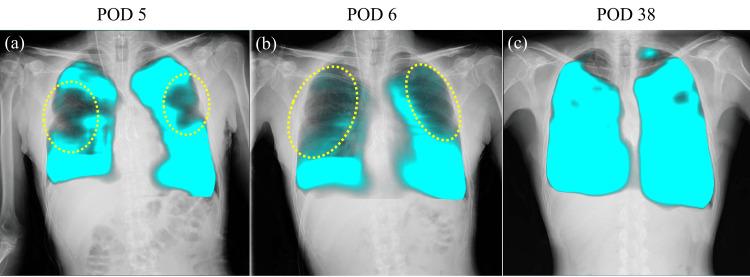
Patient’s dynamic images obtained using dynamic digital radiography (DDR) (PL-mode) In the PL-mode, the dynamic ventilation image is shown, demonstrating a blue shadow in the position where the lung tissue is expanded by breathing. Yellow dotted ellipses indicate hypoventilation. PL-mode: pixel value measurement in a low-frequency mode
